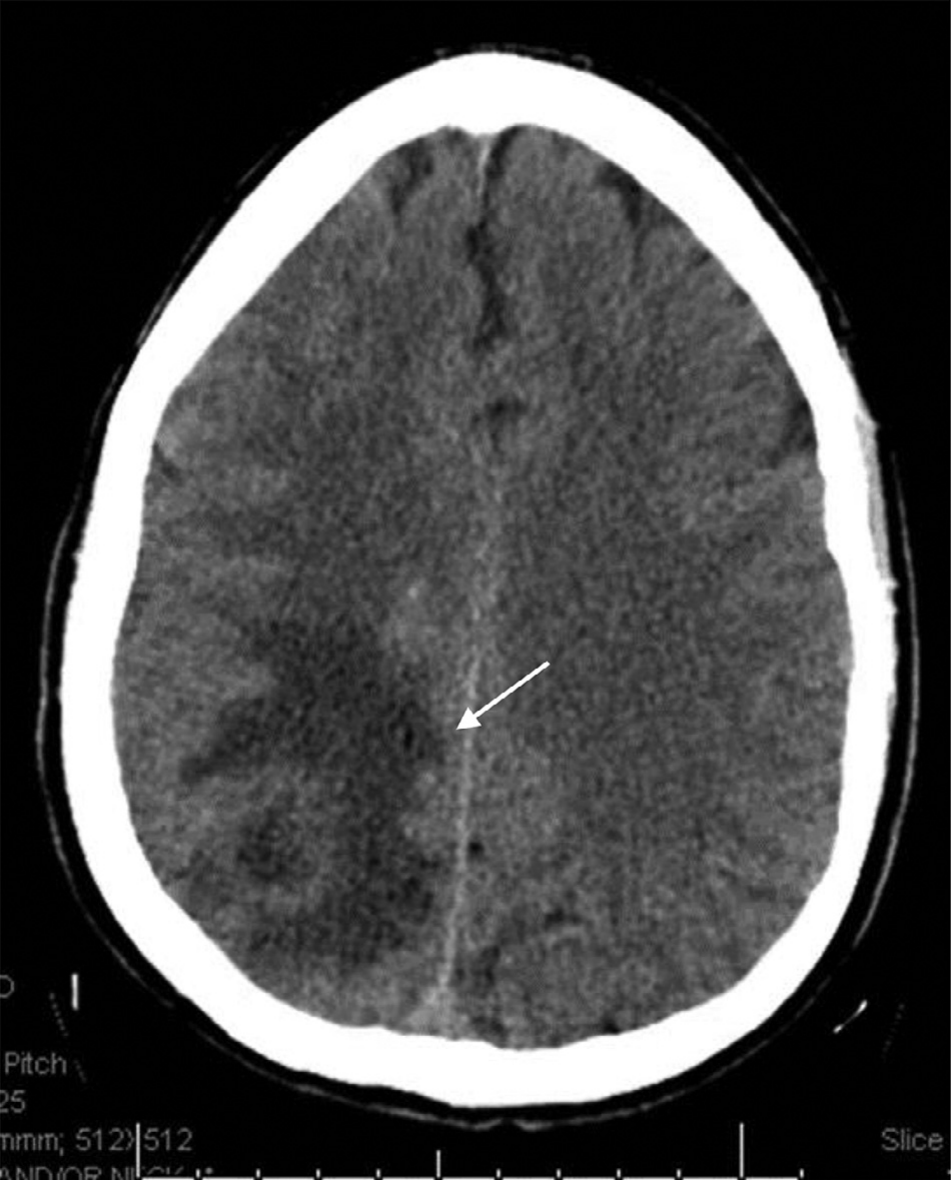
The CTA of the brain. The cut provided is a post contrast cut showing a large peripherally enhancing mass centered within the right parietal lobe measuring approximately 2.5 × 2.7 cm with surrounding vasogenic edema and localized mass-effect with a 0.6 cm right to left midline shift.
